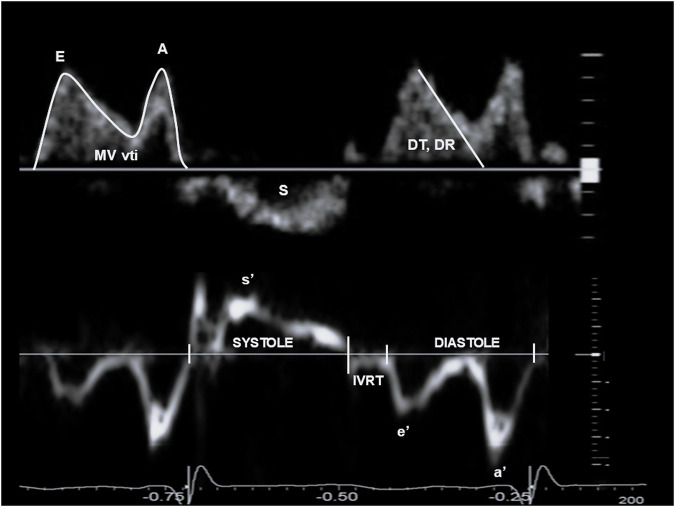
Pulse wave Doppler (top) and Tissue Doppler (bottom) trace with the various stages and physiology of the cardiac cycle and its representative ultrasound parameters. S outflow wave, MV vti mitral valve velocity time integral, E early diastolic wave, A atrial contraction wave, DT deceleration time, DR deceleration rate, s’ systolic myocardial velocity, e’ early diastolic myocardial velocity, a’ atrial contraction myocardial velocity, IVRT isovolumetric relaxation time.
